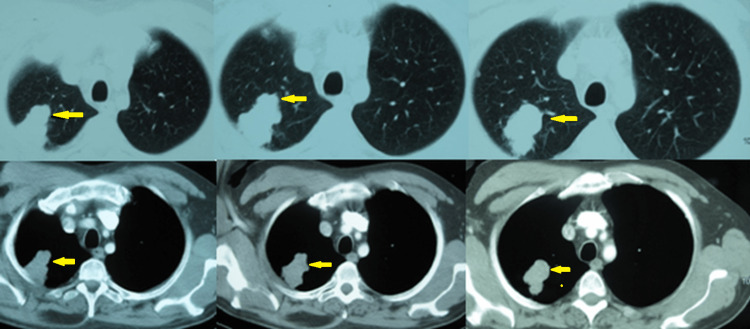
Chest computed tomography on December 31, 2007. Chest axial images (soft tissue and lung windows) revealing a mass of 6 cm × 3 cm in the right upper lobe (apical and posterior segment) of the lung without lymph nodes and some micronodules in the upper and middle lobe.
